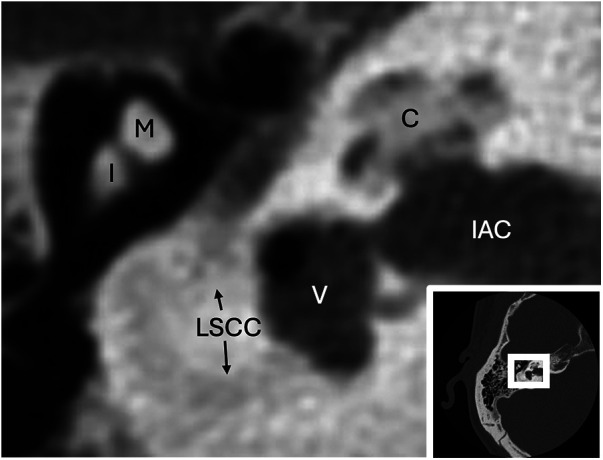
Labyrinthitis ossificans. Axial CT shows extensive ossification of the cochlea (C) and lateral semicircular canal (LSCC) due to severe labyrinthitis ossificans. Vestibule, malleus head (M), incus body (I), and internal auditory canal (IAC) are provided for reference.
